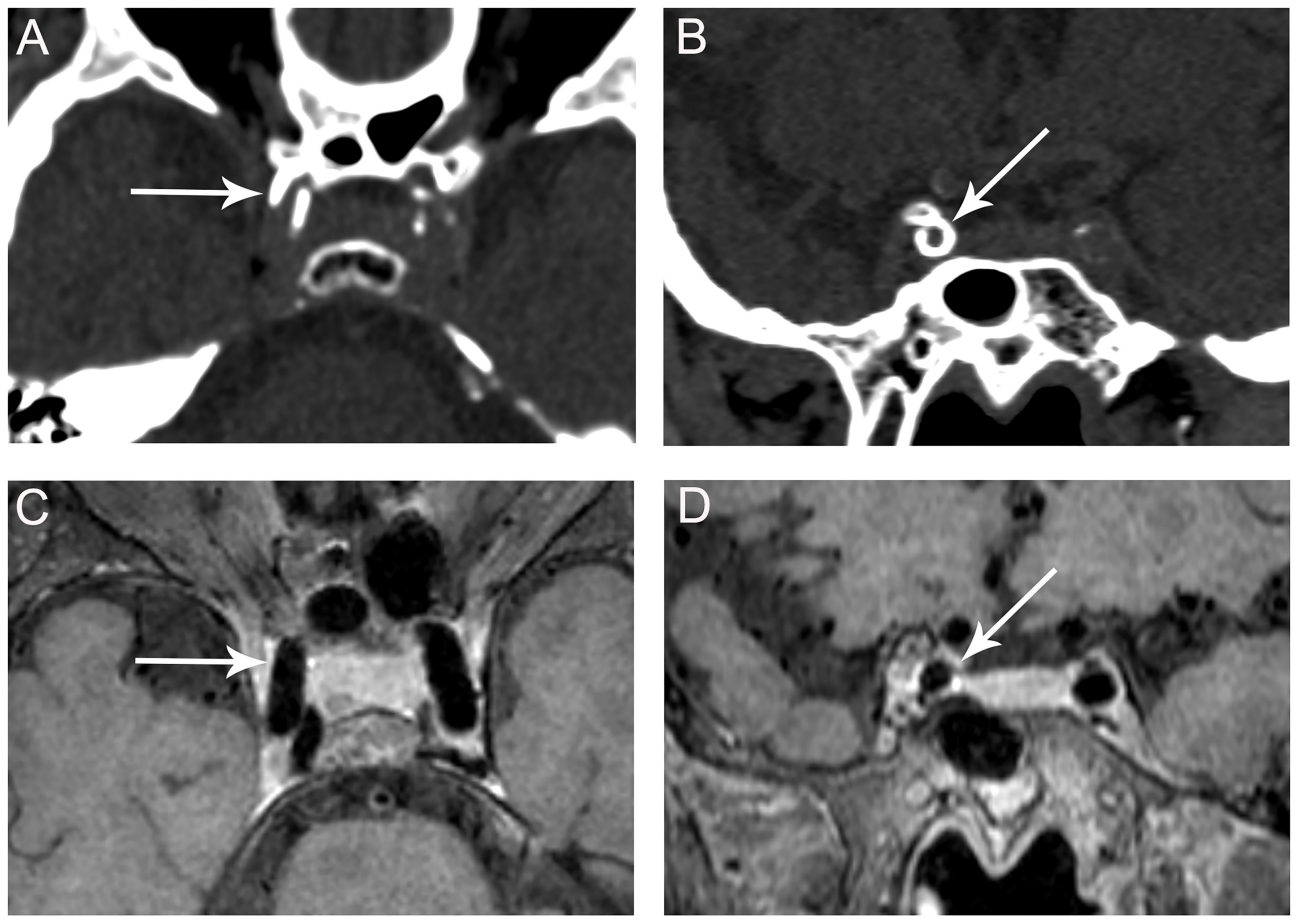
Representative images of predominant medial ICA calcification. CT shows a continuous, circumferential calcification on the long (A, arrow) and short axes (B, arrow) of the right cavernous segment of the ICA suggesting medial calcification. No corresponding plaque is observed on VWMRI (C, D, arrows).
